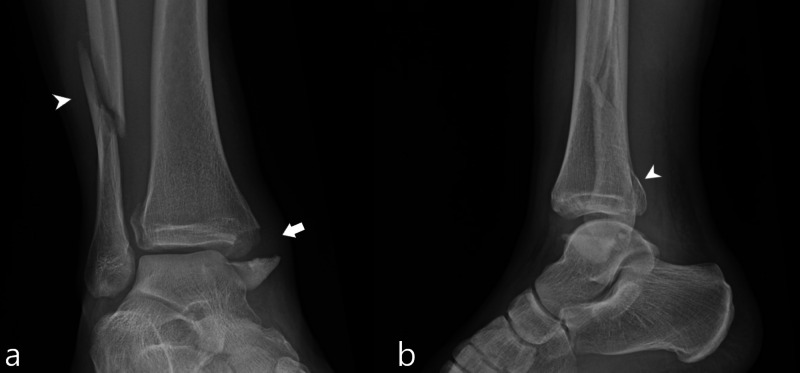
Case 9 radiographic images of the ankle A frontal radiograph of the ankle (a) demonstrates a nondisplaced fracture of the lateral malleolus (white arrowhead) and a moderately displaced fracture of the medial malleolus (white arrow). A lateral radiograph of the ankle (b) demonstrates a mildly displaced posterior malleolar fracture (white arrowhead).
